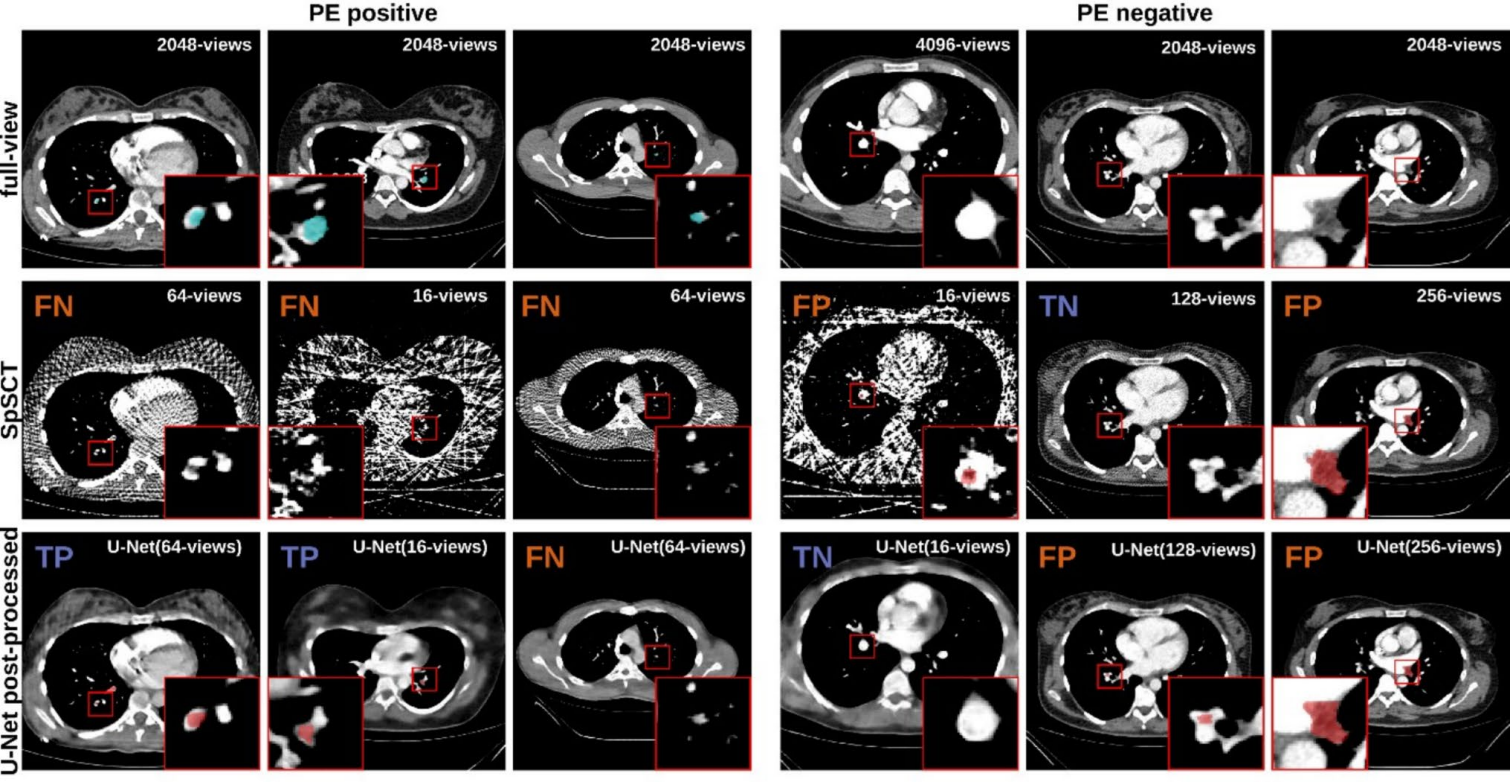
Examples of pulmonary embolism (PE) segmentations. Each column displays a full-view image, followed by its corresponding SpSCT (sparse sampling CT) reconstruction, and then the U-Net post-processed SpSCT image. The first three columns depict images labeled with PE, while the last three columns show images labeled as healthy. The gold standard segmentations are shown in the PE positive full-view images as blue; the segmentations by the readers in red. In the upper left corner of each image, it is indicated whether the images were correctly marked (true positive [TP] or true negative [TN]) or not (false negative [FN] or false positive [FP]). All images are presented in the soft tissue window [center: 60 HU, width: 360 HU]
